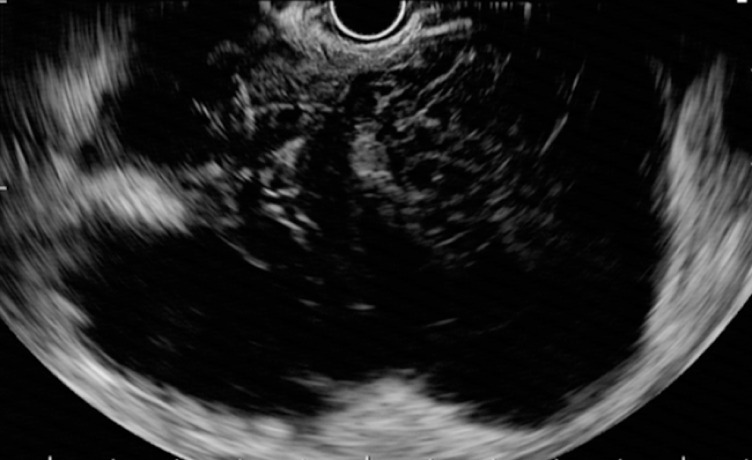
Endoscopic ultrasound image showing that the tumor connected with the gastric wall could not be detected because of hemorrhage around the stomach.
